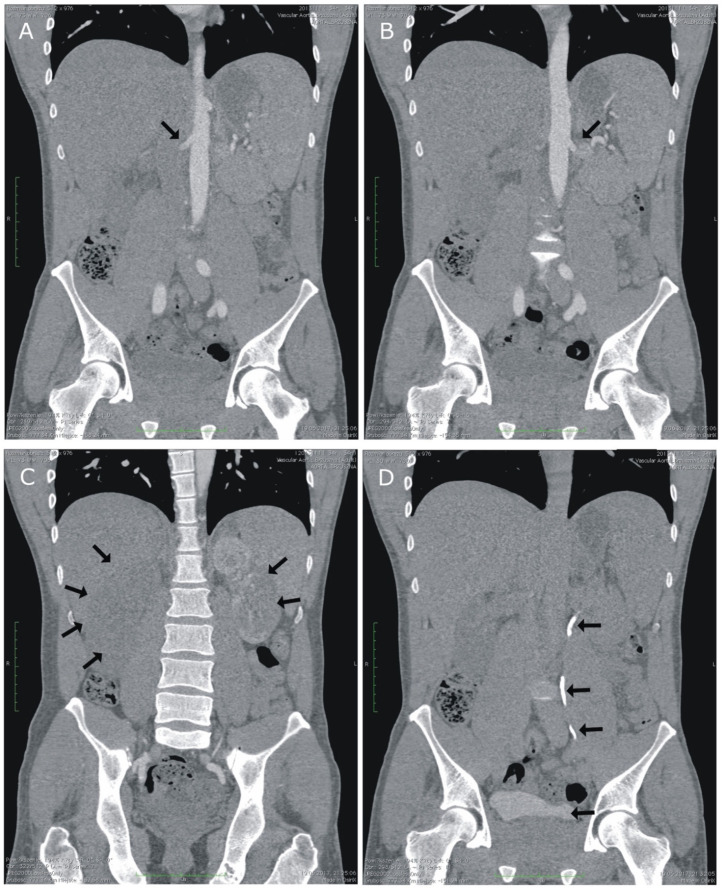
(A,B) Angio-computed tomography scans showing areas of thrombosis in the renal arteries. (C) Infarction (not enhancing) areas within the right and left kidneys. (D) Preserved excretion of contrast urine by intact portions of the left kidney parenchyma.
